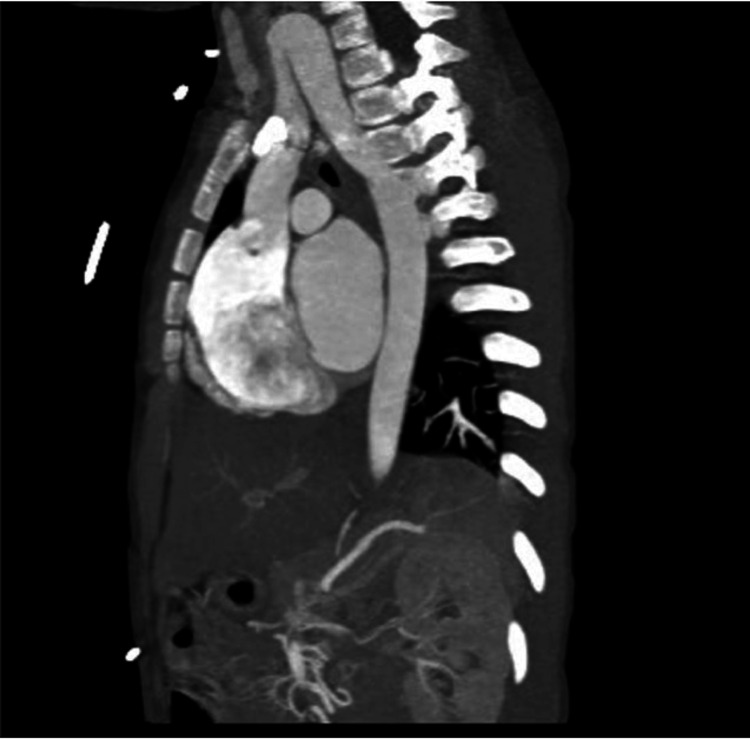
Sagittal view from chest CTA CTA, CT angiography
